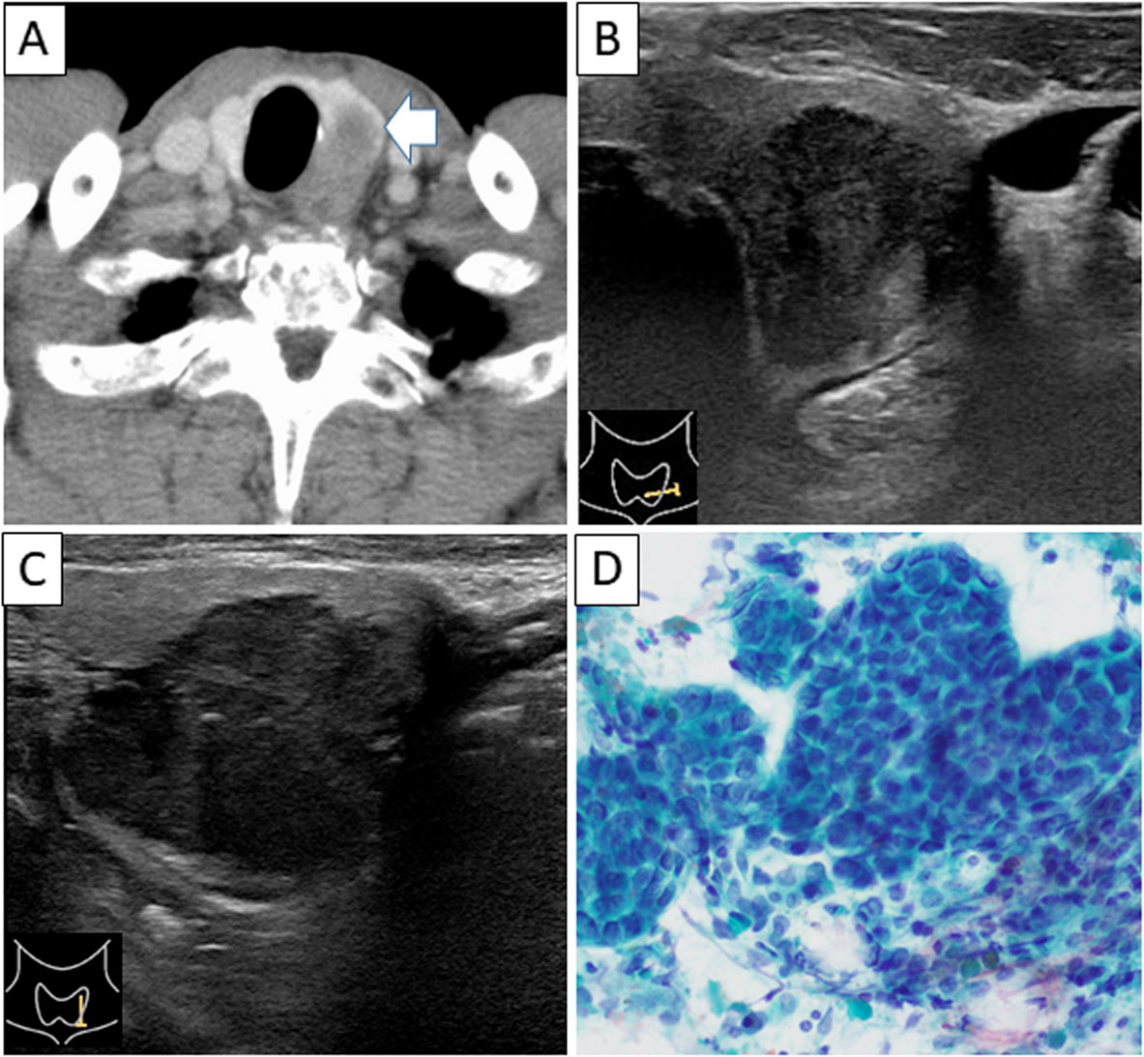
Imaging and pathological findings. a Contrast-enhanced computed tomography (CT) revealed a low-density mass in left thyroid gland. b, c Ultrasonographic (US) examination showed a hypoechoic, indistinct border and heterogeneous nodule measuring 16.9 mm × 19.7 mm × 23.9 mm in the left lobe of the thyroid gland. d Pathological examination of an ultrasound-guided fine-needle aspiration showed adenocarcinoma
